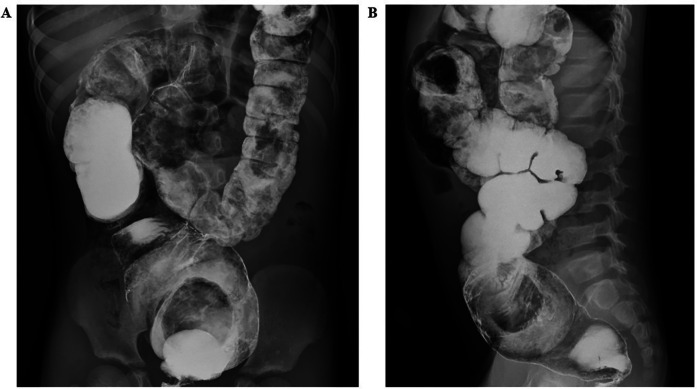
A barium enema examination (A and B are respectively the anteroposterior and lateral X-ray films of the abdomen) revealed a large filling defect about 6 cm in diameter at the junction of the sigmoid colon and rectum, almost complete obstruction of the colon, resulting in fecal retention.
